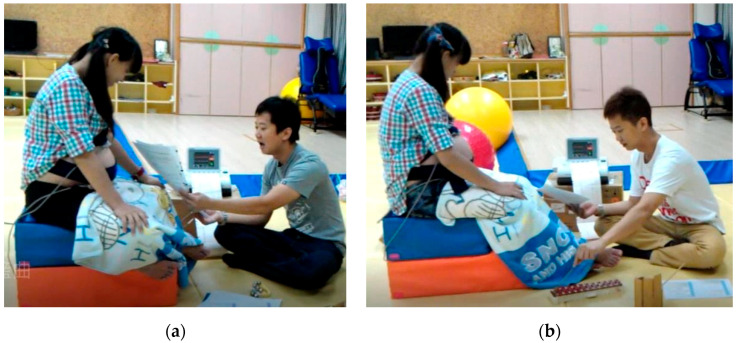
Study space and Doppler fetal monitor configuration (a) Singing Time and Chanting; (b) Musical Storytelling.
